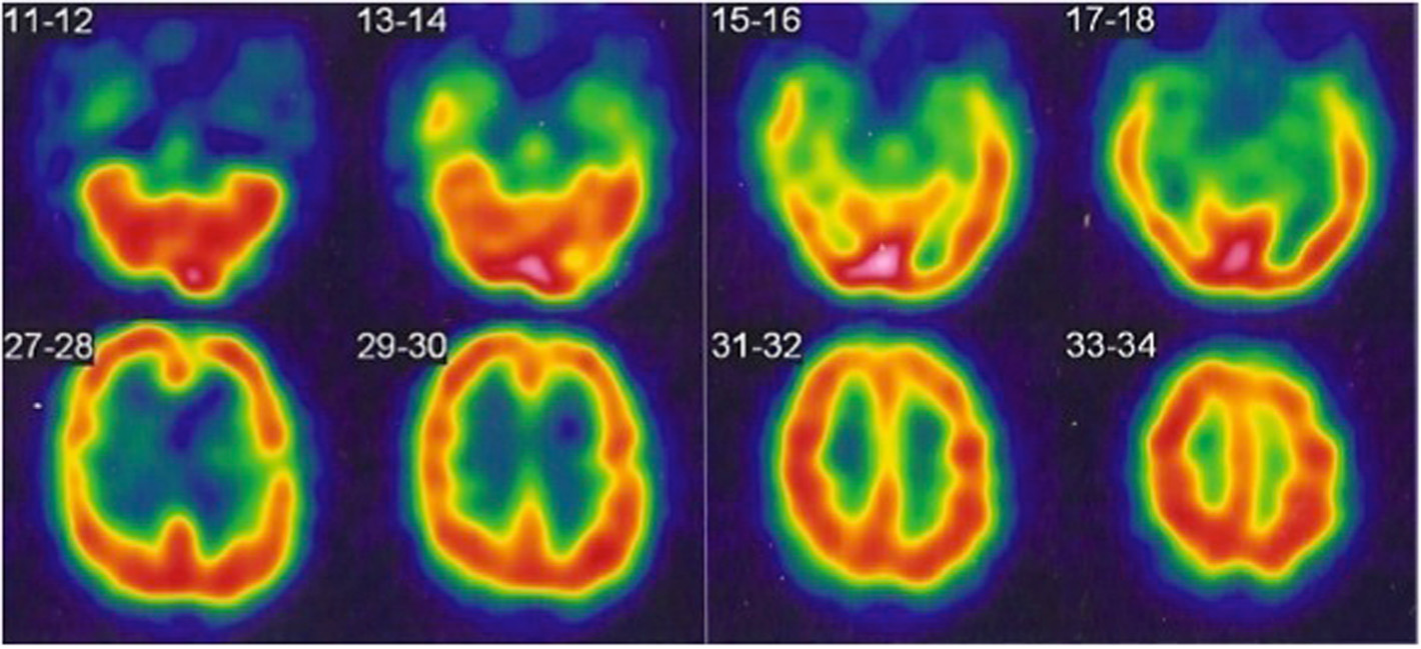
SPECT of the patient with chronic BE showing bilateral temporal and frontal hypoperfusion.
